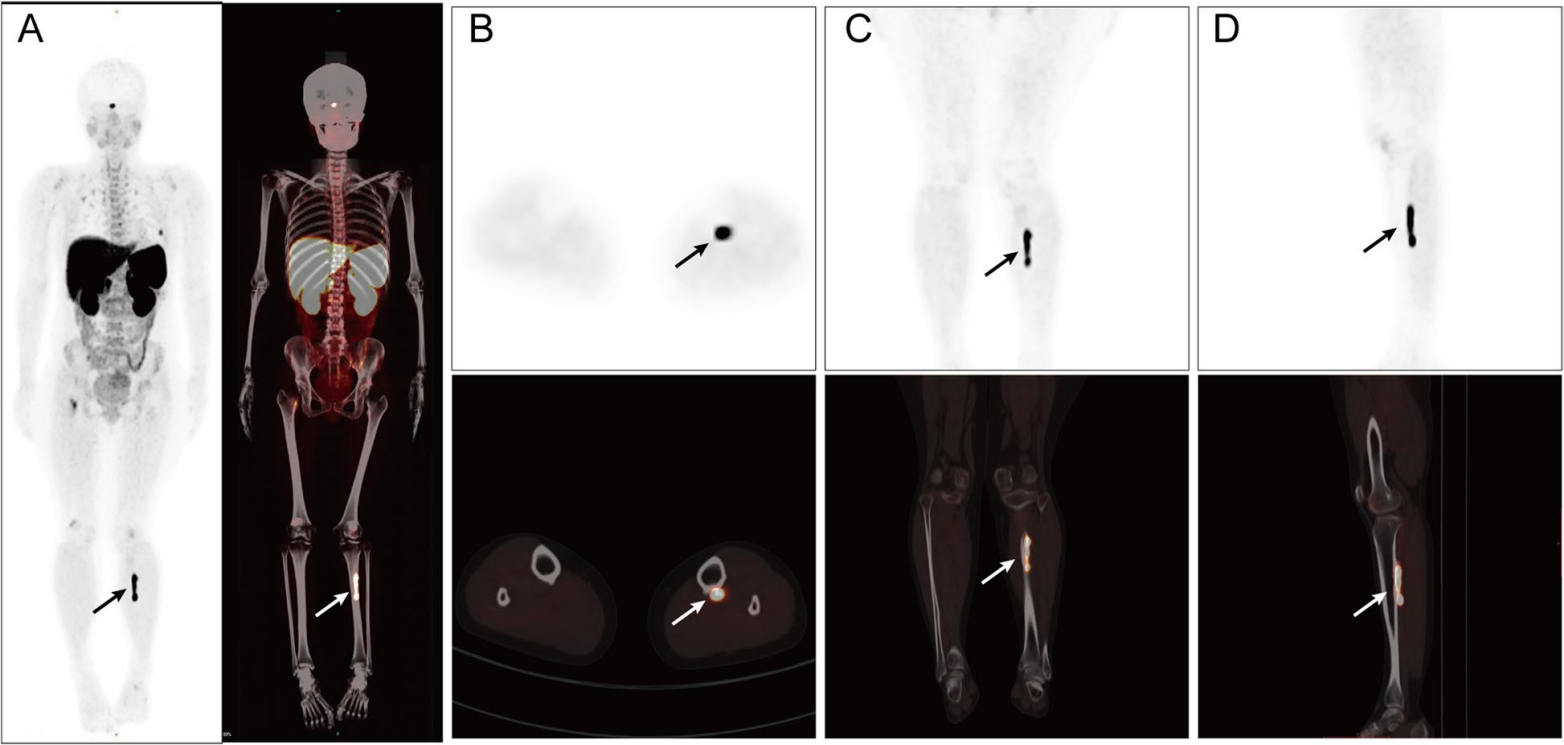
^68^Ga-DOTATATE PET/CT imaging of tumor-induced osteomalacia. ^68^Ga-DOTATATE PET/CT shows abnormal density of left middle and upper tibia with increased SSTR expression. Pseudo fracture of multiple ribs and right femoral neck with increased SSTR expression
